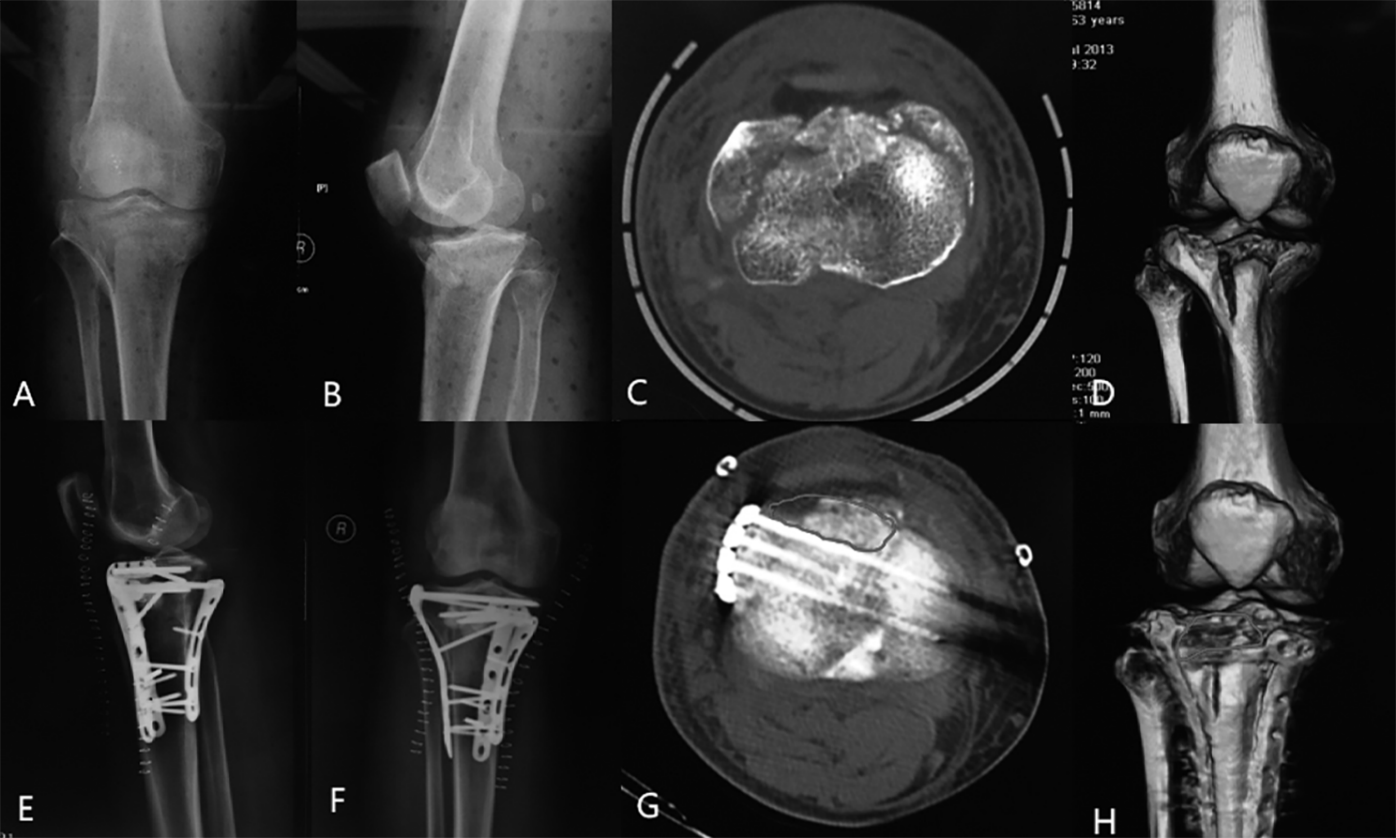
A 63-year-old male patient presented with bicondylar HTPF (A-D). Subsequently, open reduction and fixation using anteromedial and anterolateral locking plates were performed (E, F). Postoperative CT scans (G, H) revealed the “buttress bare area” behind the patella tendon (indicated by grey circles)
